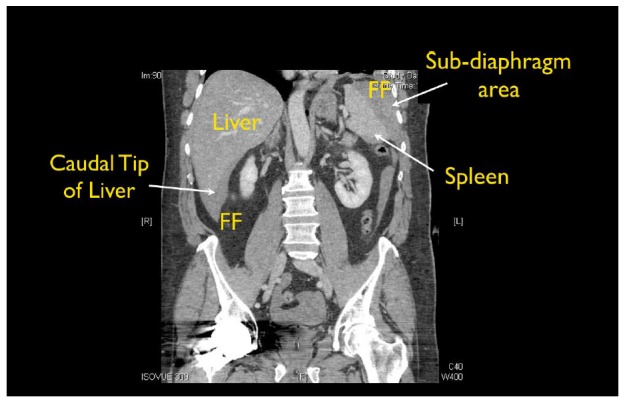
Computed tomography showing accumulation of free fluid (FF) in a traumatic supine patient.
